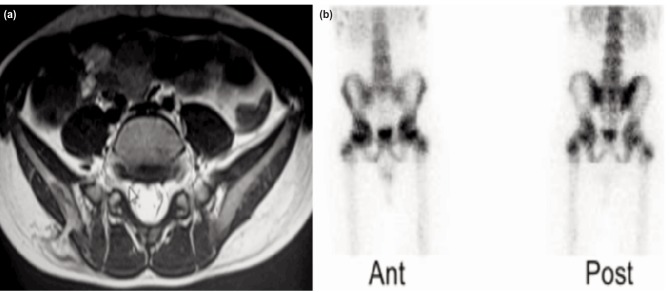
MRI-2 hyper-intense foci; intramedullary and in subcutaneous plane with enhancement 99mTc-MDP scan increased tracer uptake in the right posterior ilium.
